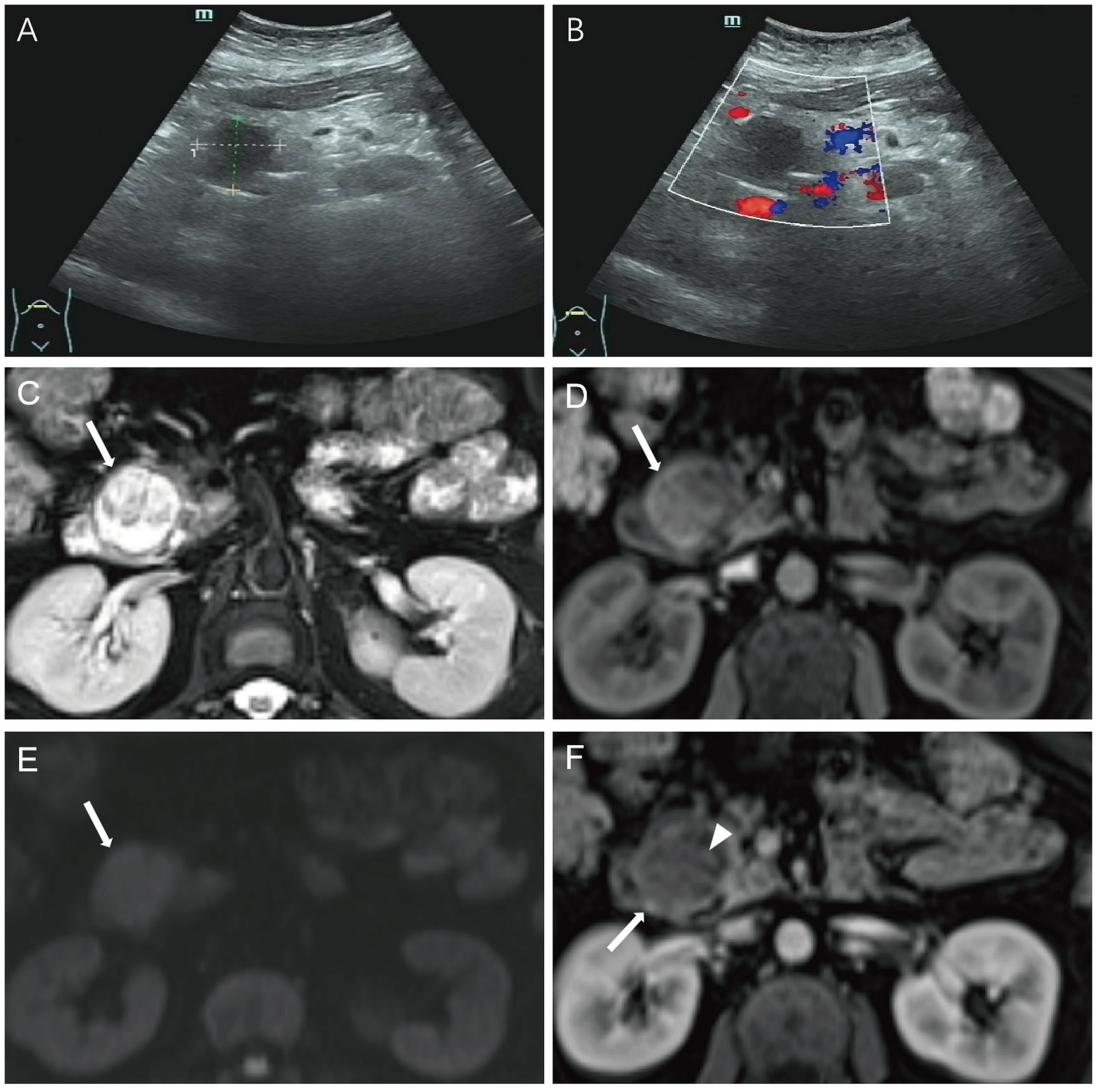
Case 1: abdominal ultrasound (US) revealed a ‌hypoechoic mass lesion anterior to the pancreatic head‌ (A,B). Axial T2 Flair (C) and T1WI (D) showed a well-defined and mixed signal intensity lesion in the pancreatic head (white arrow). The ‌solid component‌ was hyper-intense on DWI‌ (E). In the arterial phases (F), the lesion demonstrated peripheral enhancement (white arrow), with scattered patchy delayed enhancement internally (arrowhead).
